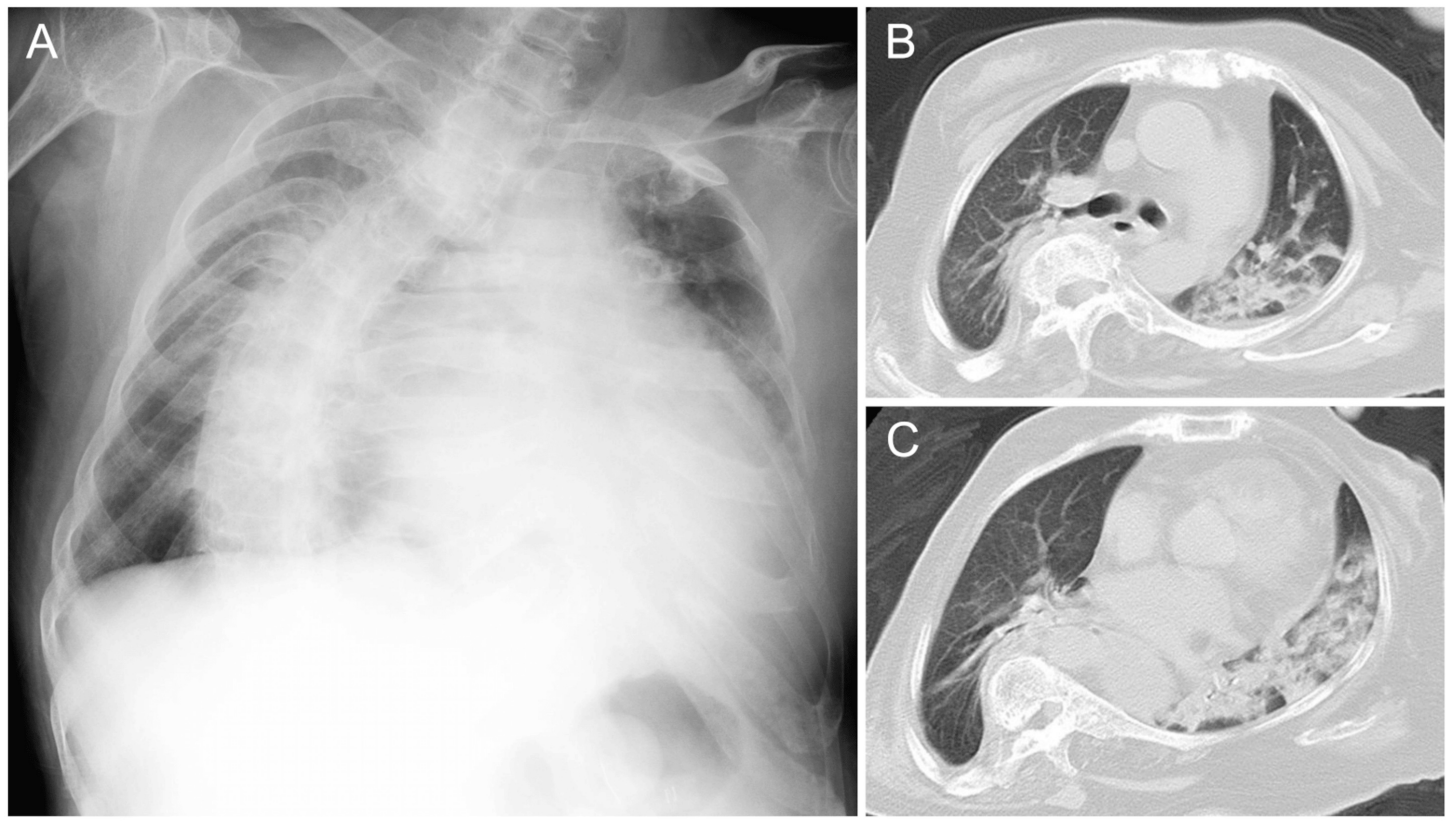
Chest X-ray and CT findings. (A) Chest X-ray showed cardiomegaly, left pulmonary infiltration, and scoliosis at the onset of illness. (B)-(C) CT demonstrated infiltration in both the left upper and lower pulmonary fields consistent with aspiration pneumonia at the onset of illness. CT: computed tomography
